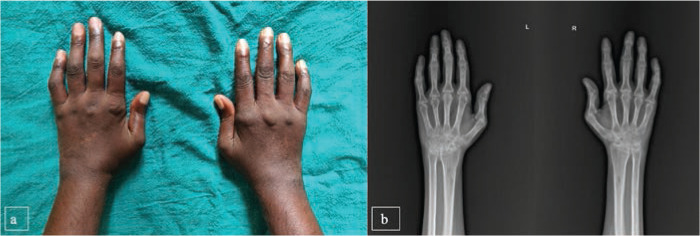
(A) bilateral sclerodactyly and Z-deformity of the thumbs with wrist swelling. (B) X-ray of both hands showing joint space narrowing at MCP, PIP, DIP with articular surface erosions and Z-deformity of the thumb.
